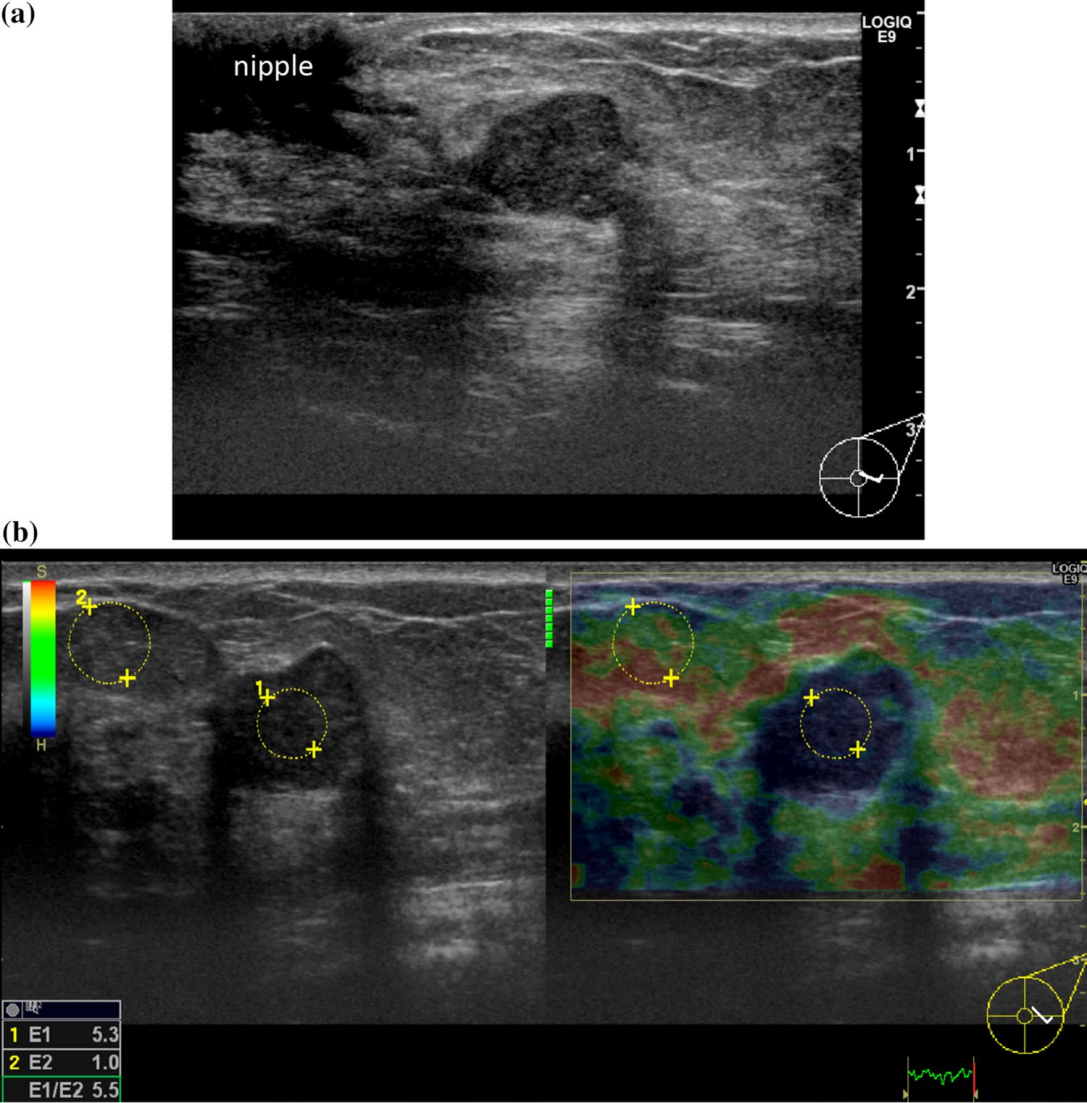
Intraductal papilloma. a On B-mode ultrasound, there is a polygonal hypoechoic tumor near the nipple and the back echo is intensified. b Strain is reduced throughout the tumor. E-index is 5.3 and E-ratio is 5.5, both of which are higher than the cut-off value
